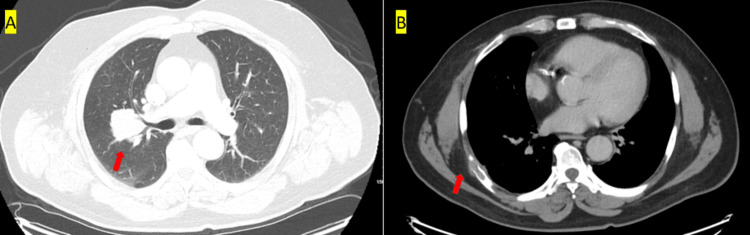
CT chest with contrast (A) 4.8 x 4.2 x 4.6 cm lobulated solid mass right upper lobe contiguous with the pulmonary hila. (B) Lytic lesion posteriorly right 7th rib with pathologic fracture.
